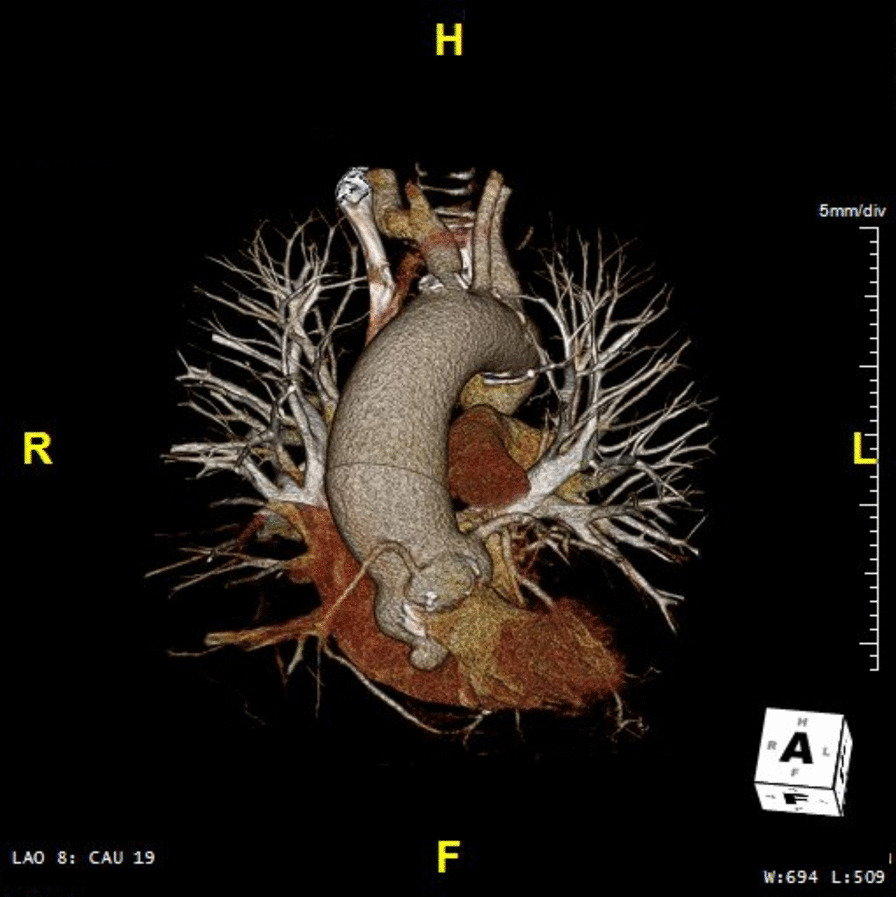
3D CT-scan reconstructions
